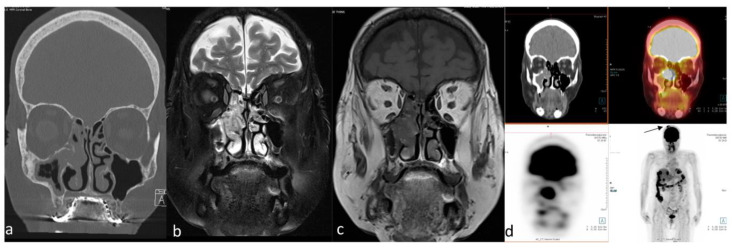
Sinonasal involvement by lymphoma. An 84-year-old female patient with a past medical history of rheumatoid arthritis presenting with an ulcerative scalp lesion. The biopsy of the scalp lesion was consistent with EBV (+) DLBCL. Paranasal sinus CT (a) shows a right nasal cavity lesion expanding the right ostiomeatal unit and extending into the right maxillary sinus. Post-obstructive frothy secretions in the right maxillary sinus are noted. Fat-saturated coronal T2w (b) and non-fat-saturated coronal T1w (c) images better depict the primary lesion as mildly T2w hyperintense areas, whereas mucosal disease is more T2w bright. ^18^F-FDG PET/CT images (d) demonstrate the intense avidity of the right nasal cavity lesion as well as the scalp lesion (arrow) and two FDG-avid right pulmonary lesions.
